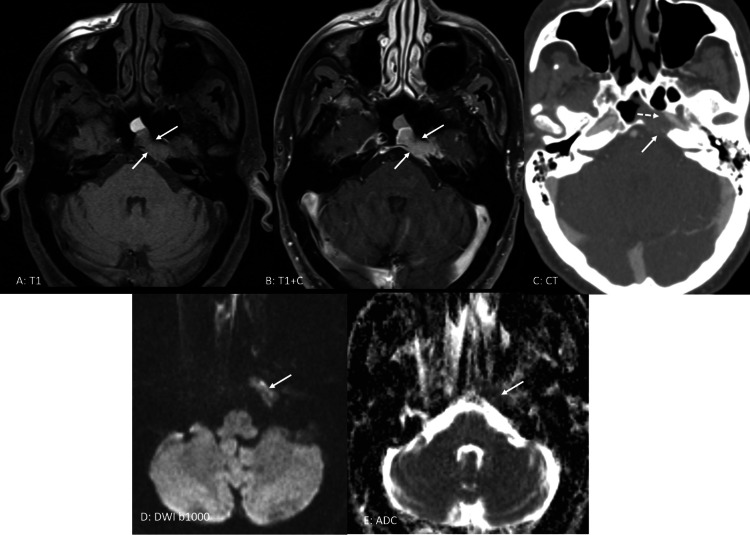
Images of skull base Pre-contrast (A) and post-contrast (B) axial T1-weighted images of the skull base demonstrate a mildly expansile, homogeneously enhancing mass (solid arrows) in the left side of the clivus in the expected location of the Dorello’s canal, the osteofibrous canal in the petrous apex containing the abducens nerve. Axial contrast-enhanced CT image (C) demonstrates an enhancing mass causing lytic destruction of bone in the clivus and petrous apex (solid arrow) with the erosion of the carotid canal (dashed arrow). Diffusion-weighted image (DWI) (D and E) demonstrates low signal within the lesion on apparent diffusion coefficient (ADC) map images (arrows) representing restricted diffusion.
